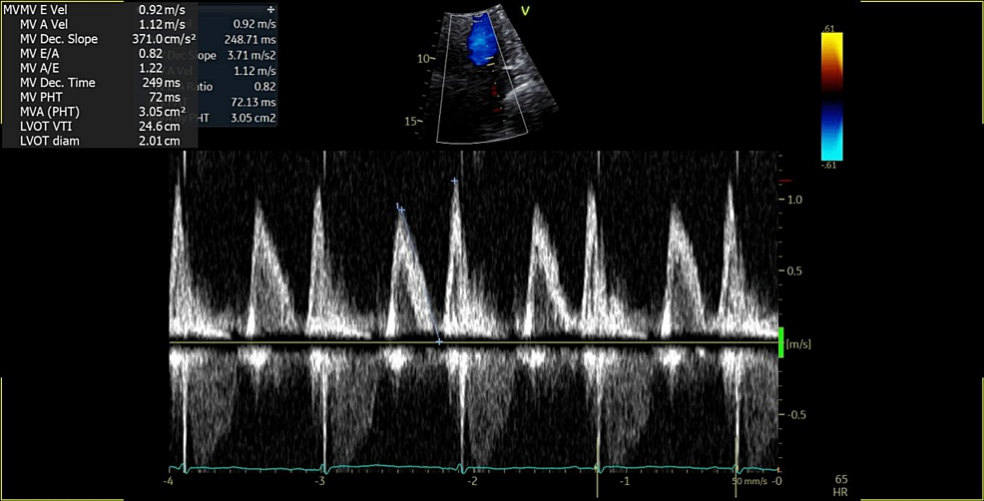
Pulse wave doppler across the mitral valve showing normal transmitral flow without any obstruction
